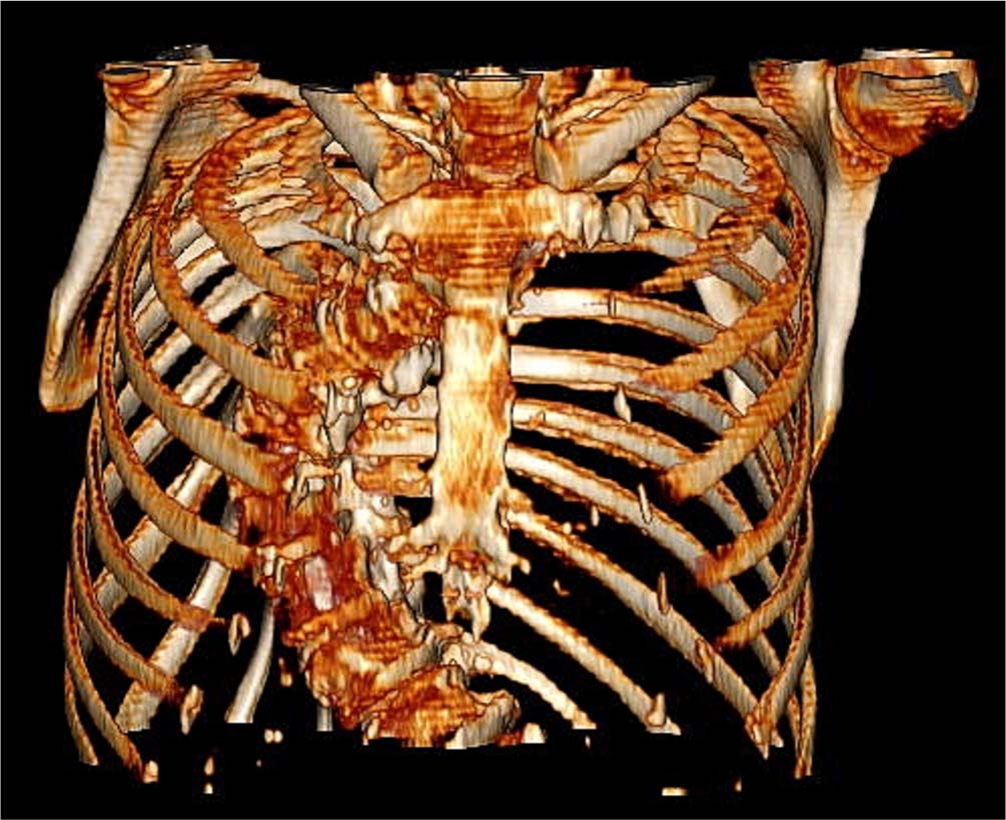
Pre-operative 3D reconstruction of patients thorax from CT.
